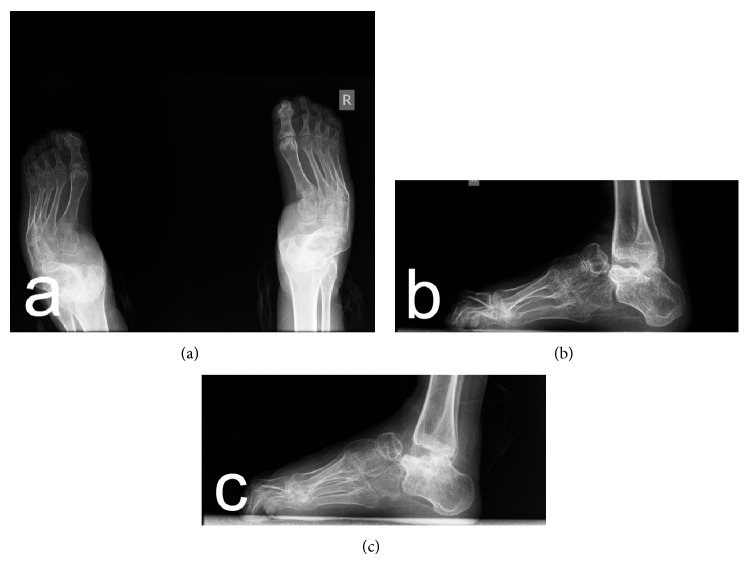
Postoperative anteroposterior (a) and lateral (b, c) radiographs of the patient (sister) with hereditary motor and sensory neuropathy type I.
